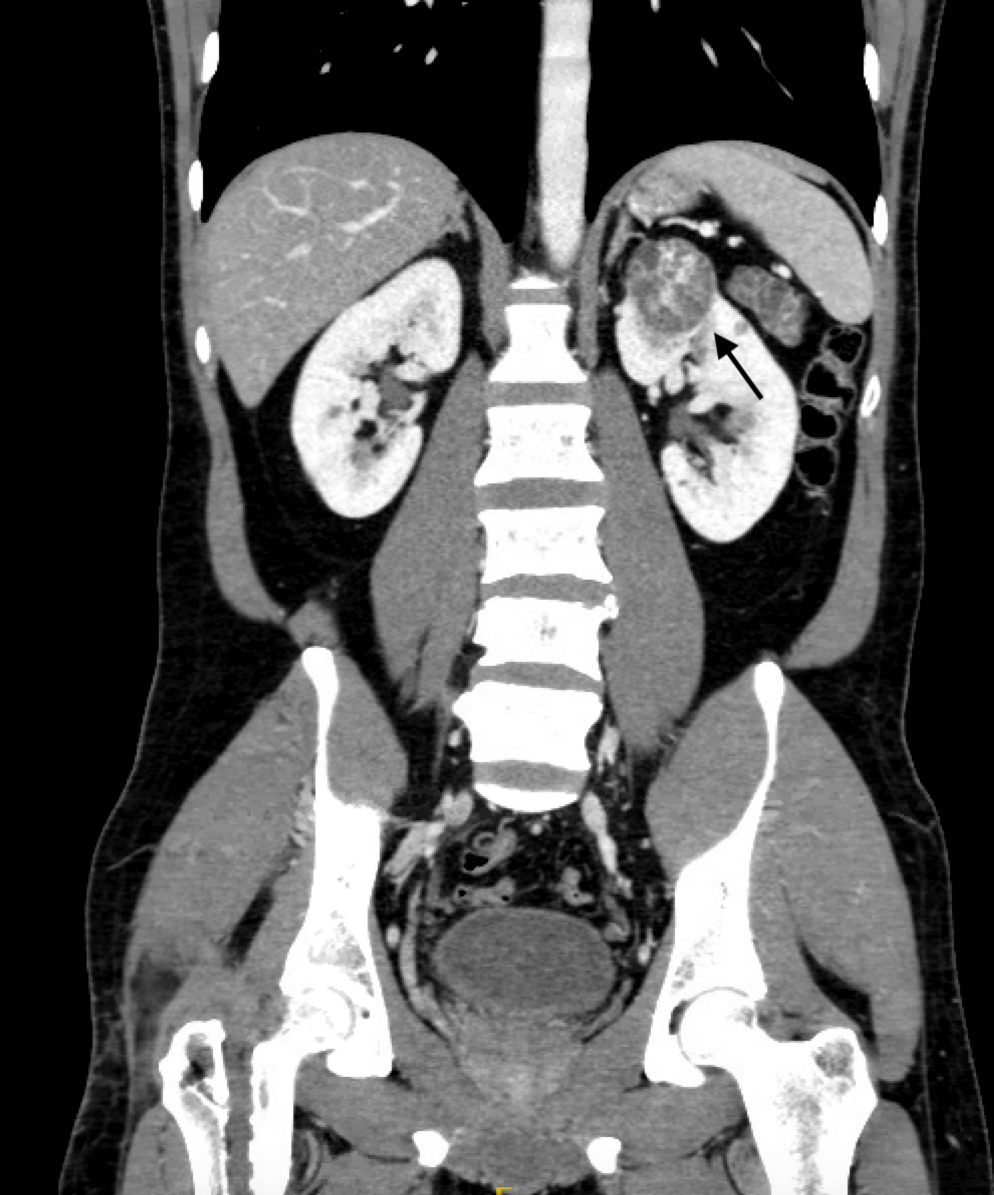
CT urography showing three complexes of multifocal RCC masses (coronal view) CT: computed tomography, RCC: renal cell carcinoma
